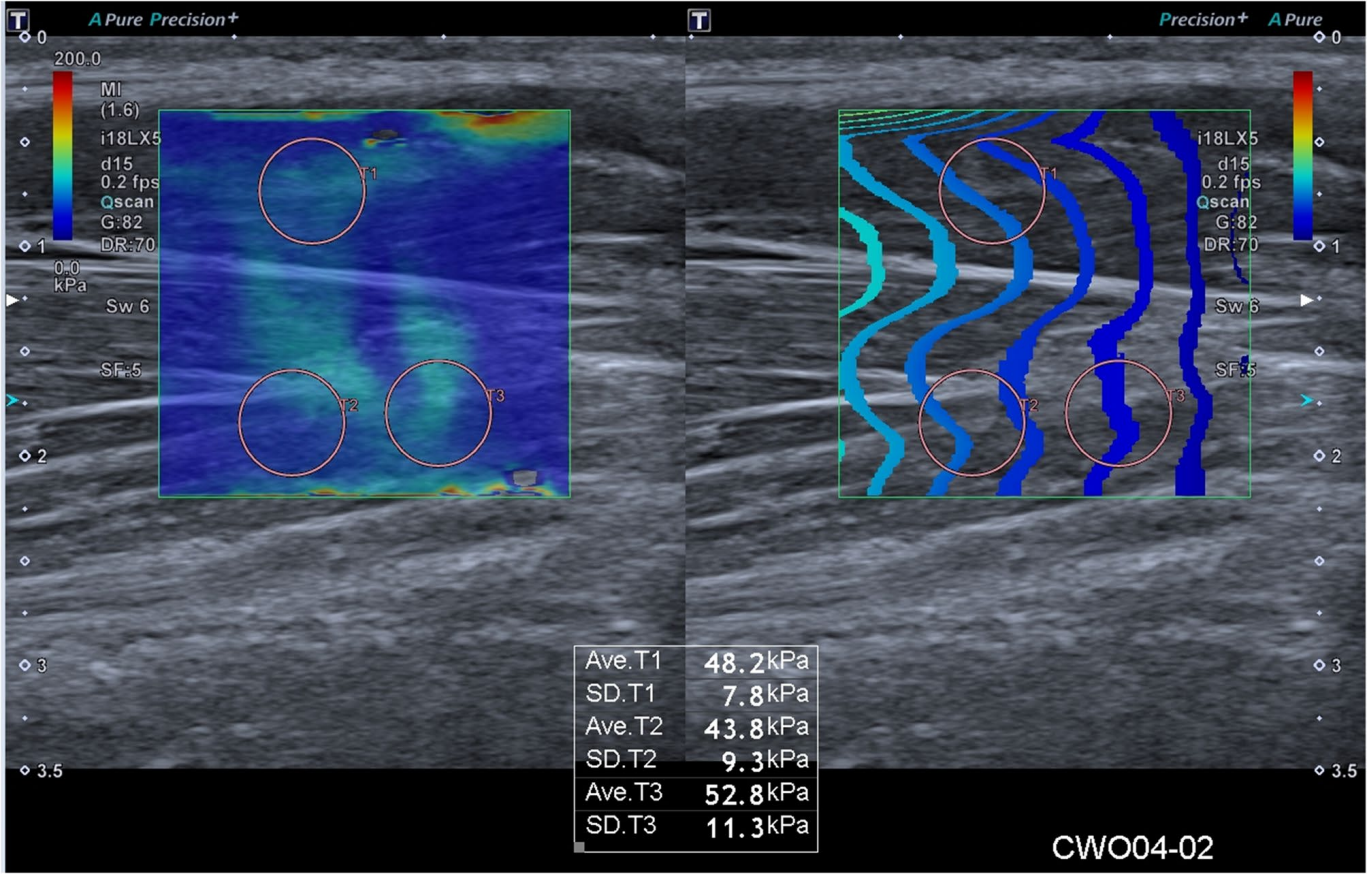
Shear wave elastography image in the region of interest with the calculated value from the ultrasound machine.
